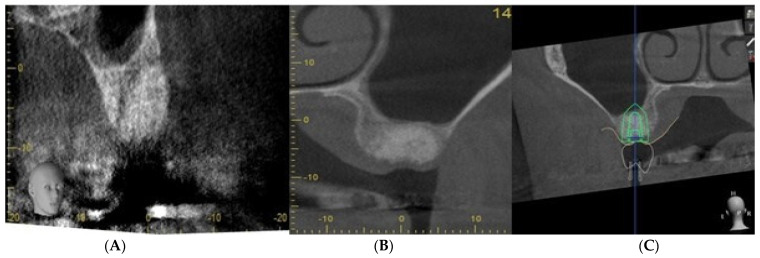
CBCT sections, (A–C) showing ridge preservation after SA.
